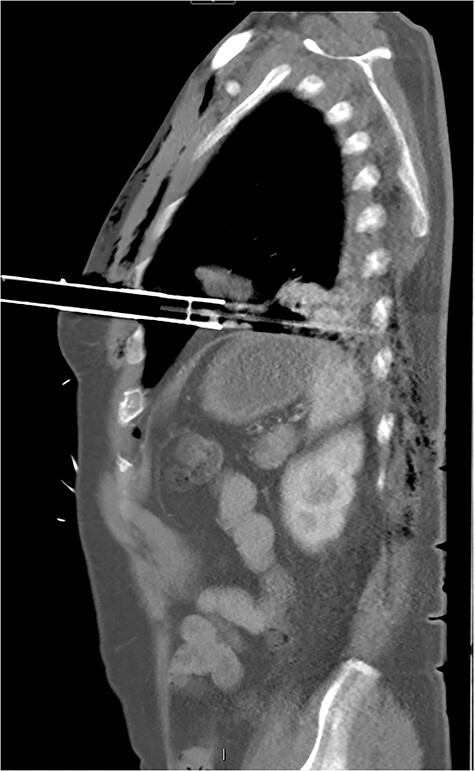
CT sagittal image showing depth of intrathoracic penetration by solar powered garden light.
